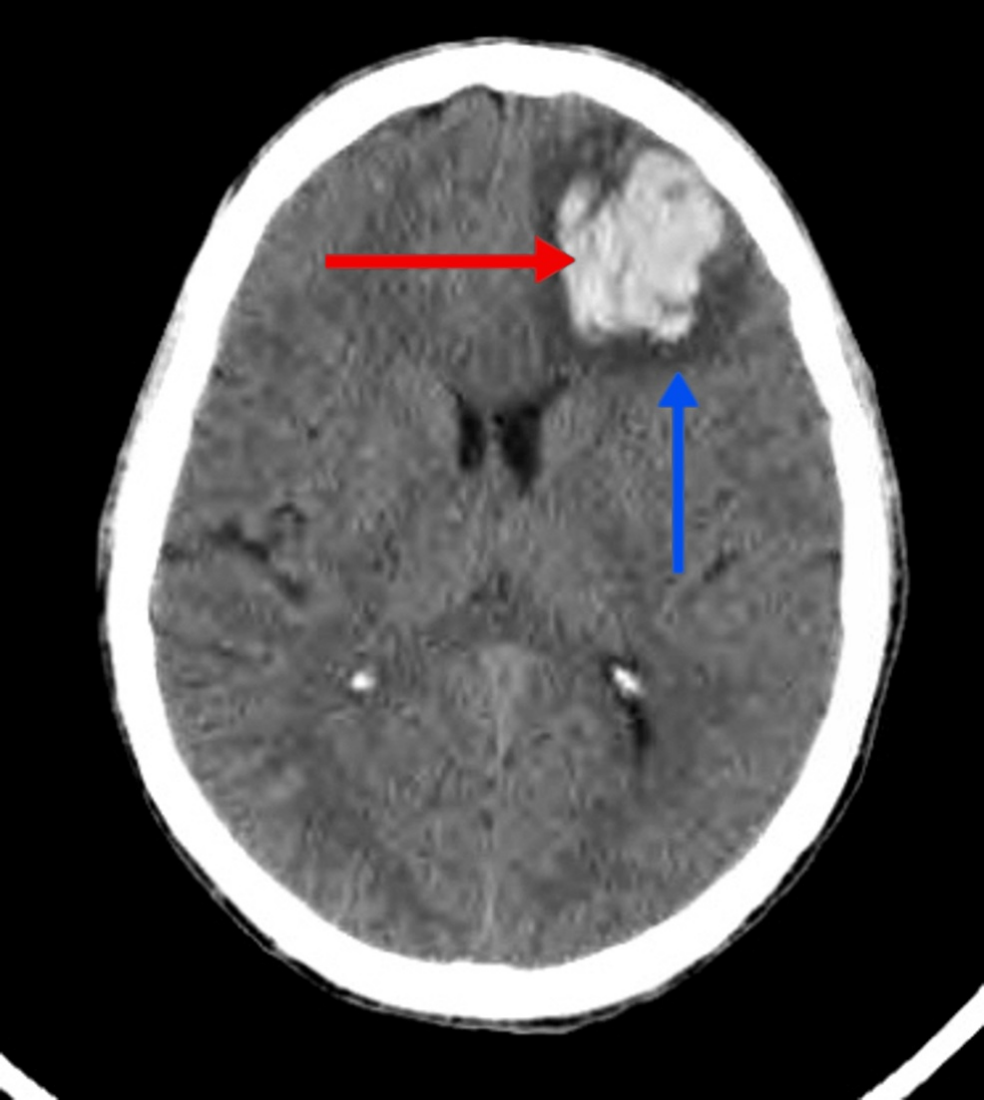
A computerized tomography (CT) scan of the head showing a large hemorrhagic focus throughout the left frontal lobe (red arrow) with a large amount of surrounding edema (blue arrow) creating mass effect upon the adjacent frontal horn of the left lateral ventricle.
